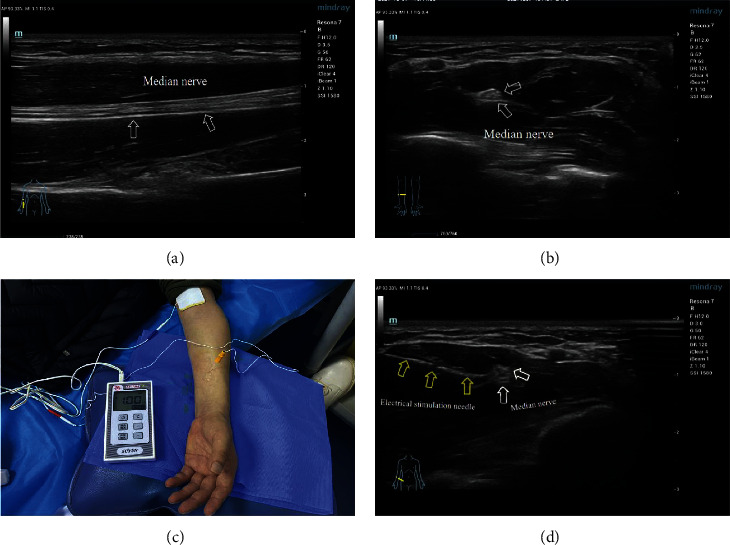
Median nerve electrical stimulation under ultrasound: (a) median nerve imaging of longitudinal section, (b) median nerve imaging of transection, (c) the ultrasound-guided median nerve electrical stimulation, and (d) UG-MNES ultrasound image. The white arrow points to the median nerve and the yellow arrow points to the electrical stimulation needle.
